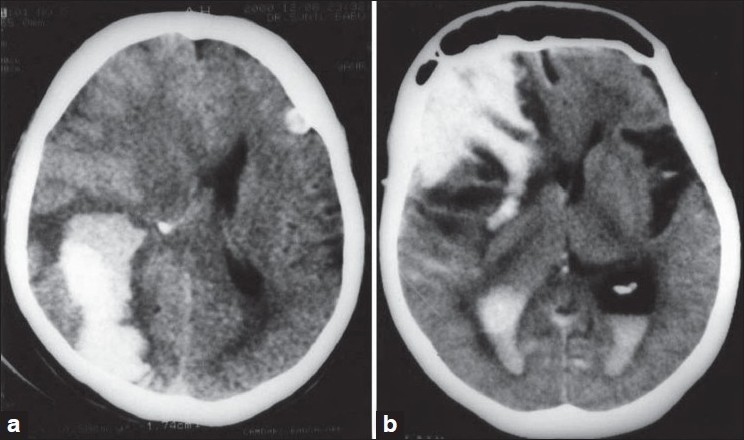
CT scans demonstrating (a) Case 1: Acute lobar hematoma in the right occipital lobe. There is evidence of mild perilesional edema and mass effect with effacement of ipsilateral lateral ventricle and midline shift. Another small cortical bleed is seen in the left frontal lobe. (b) Case 2: Acute right frontal hematoma with intraventricular extension. Perilesional edema is noted with midline shift
